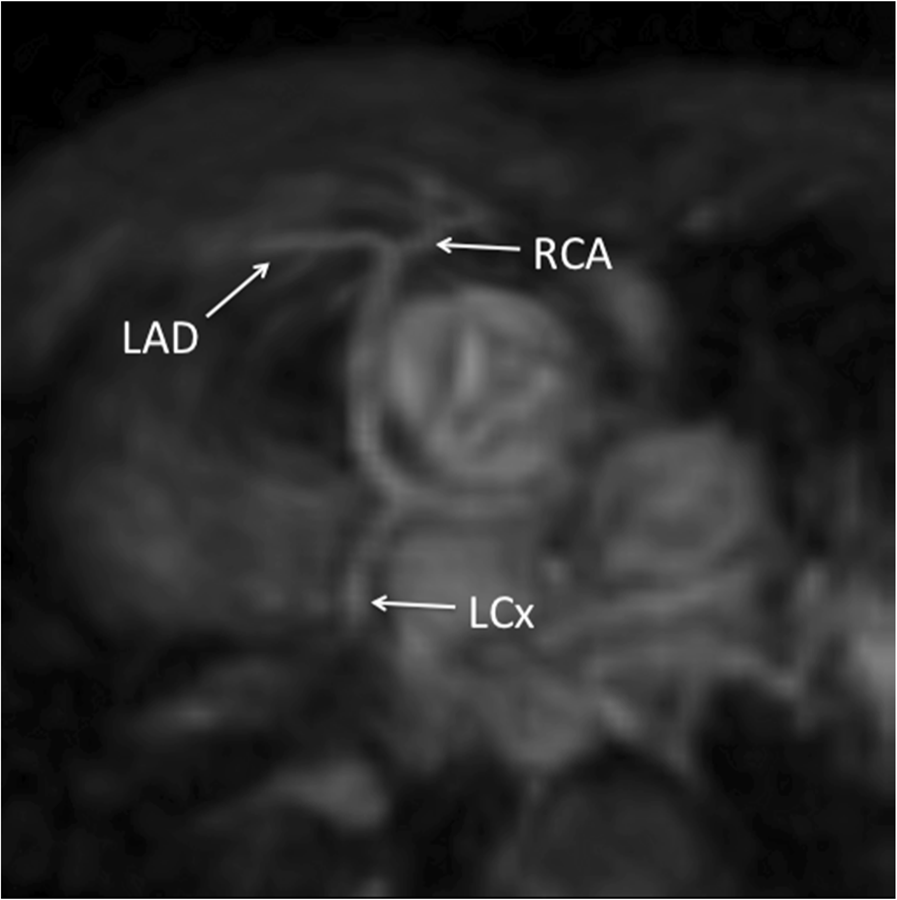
3-year old girl with Dextrocardia and Tricuspid Atresia. Single Coronary artery imaged with MRA in systole. Echocardiographic data corresponded with MRA findings
